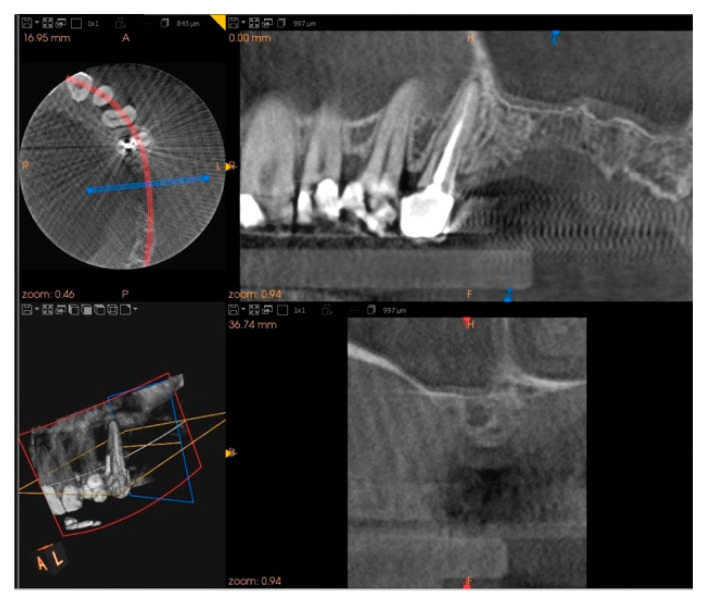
Cone-beam computed tomography (CBCT) shows bone volume preservation in the distal surface of the alveolus.
